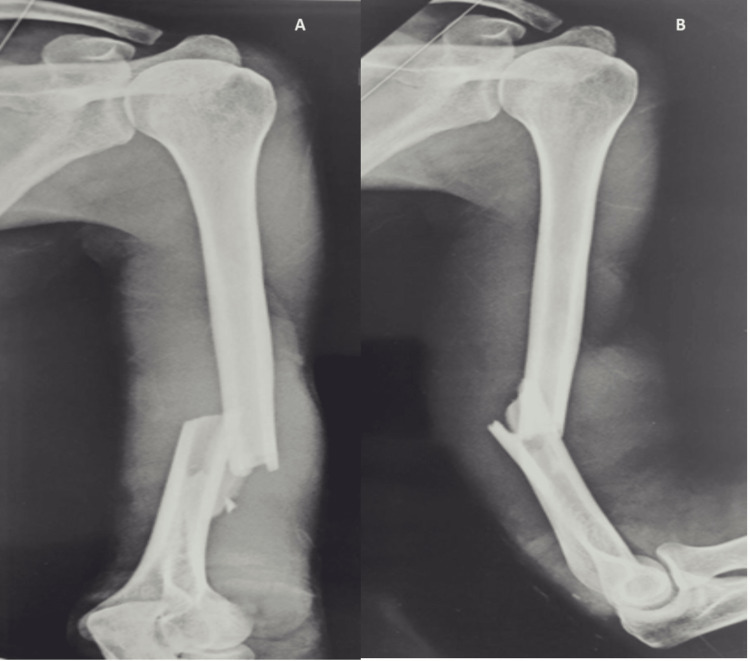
Radiograph of shaft humerus fracture (A) Anteroposterior view; (B) lateral view
